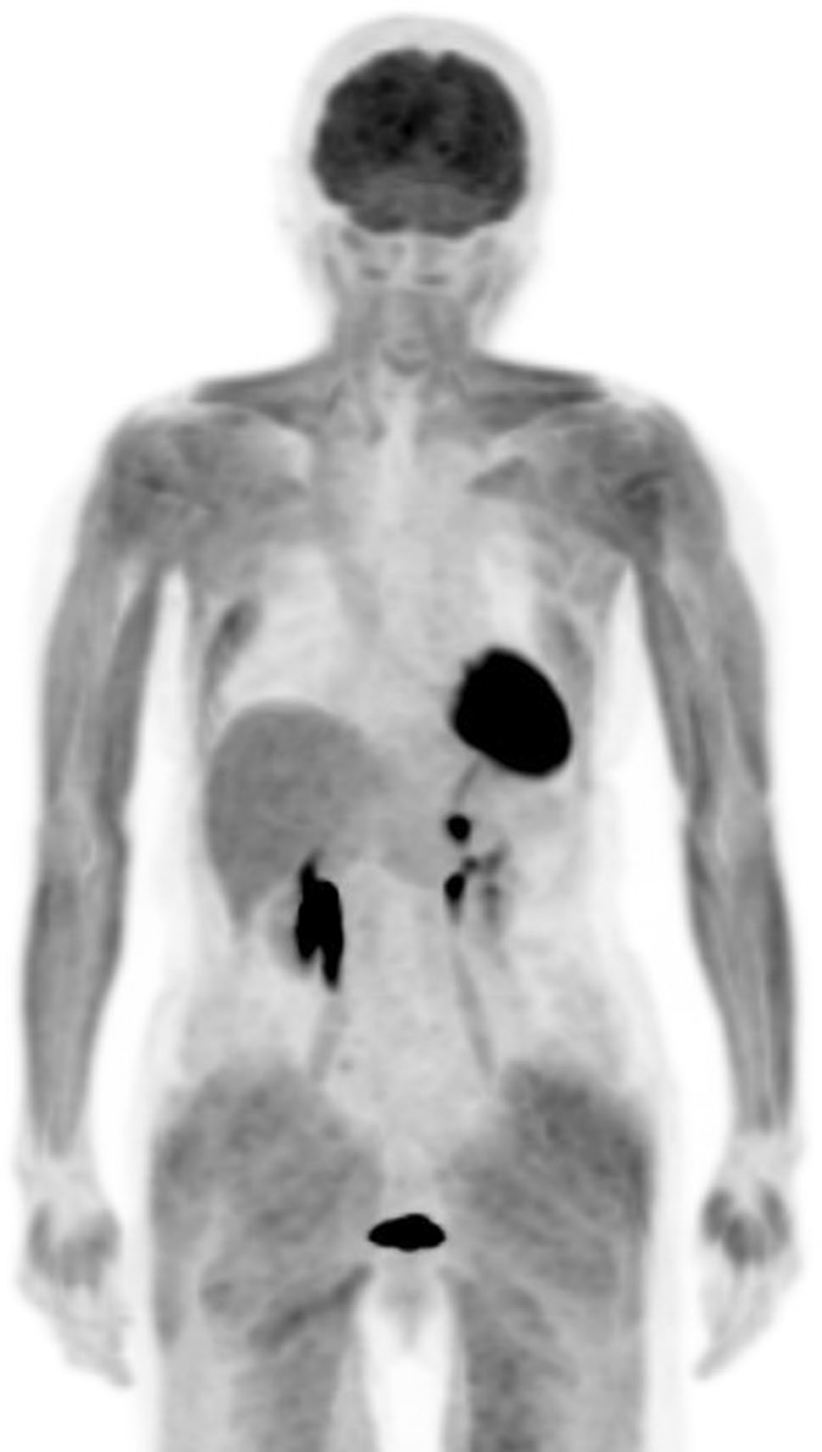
Diffuse increased accumulation in the muscles of a patient who had consumed a meal 2 h before FDG injection. An insulin injection before FDG administration causes a similar image
